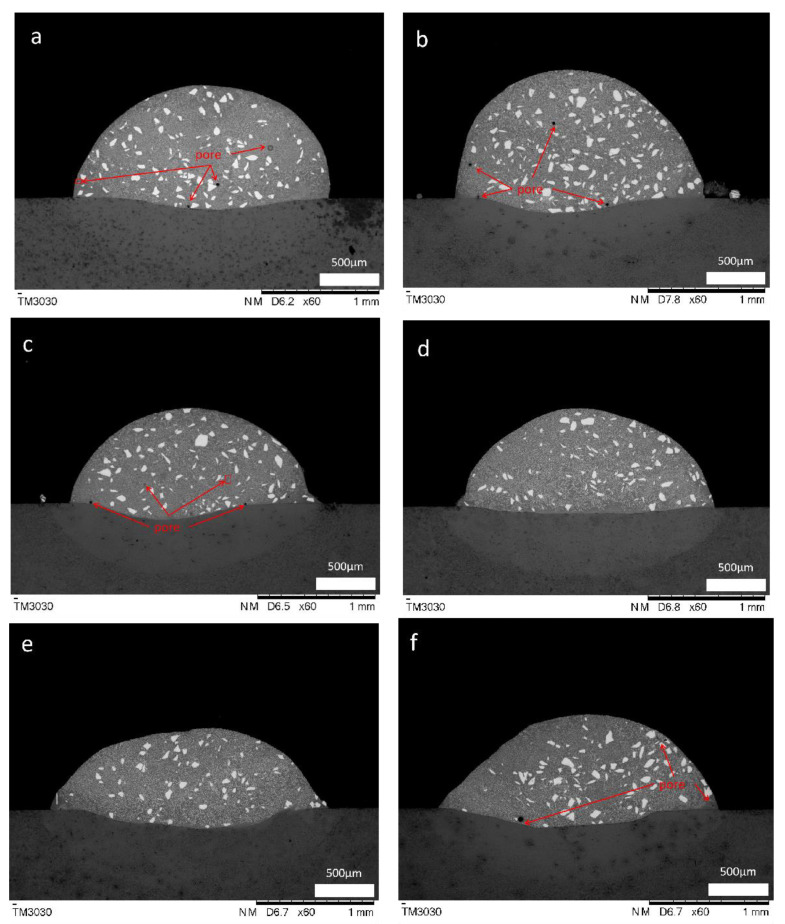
Cross-sectional morphology of the cladding layer with different ultrasonic frequencies applied: (a) 0 kHz, (b) 22 kHz, (c) 27 kHz, (d) 32 kHz, (e) 37 kHz, (f) 42 kHz.
